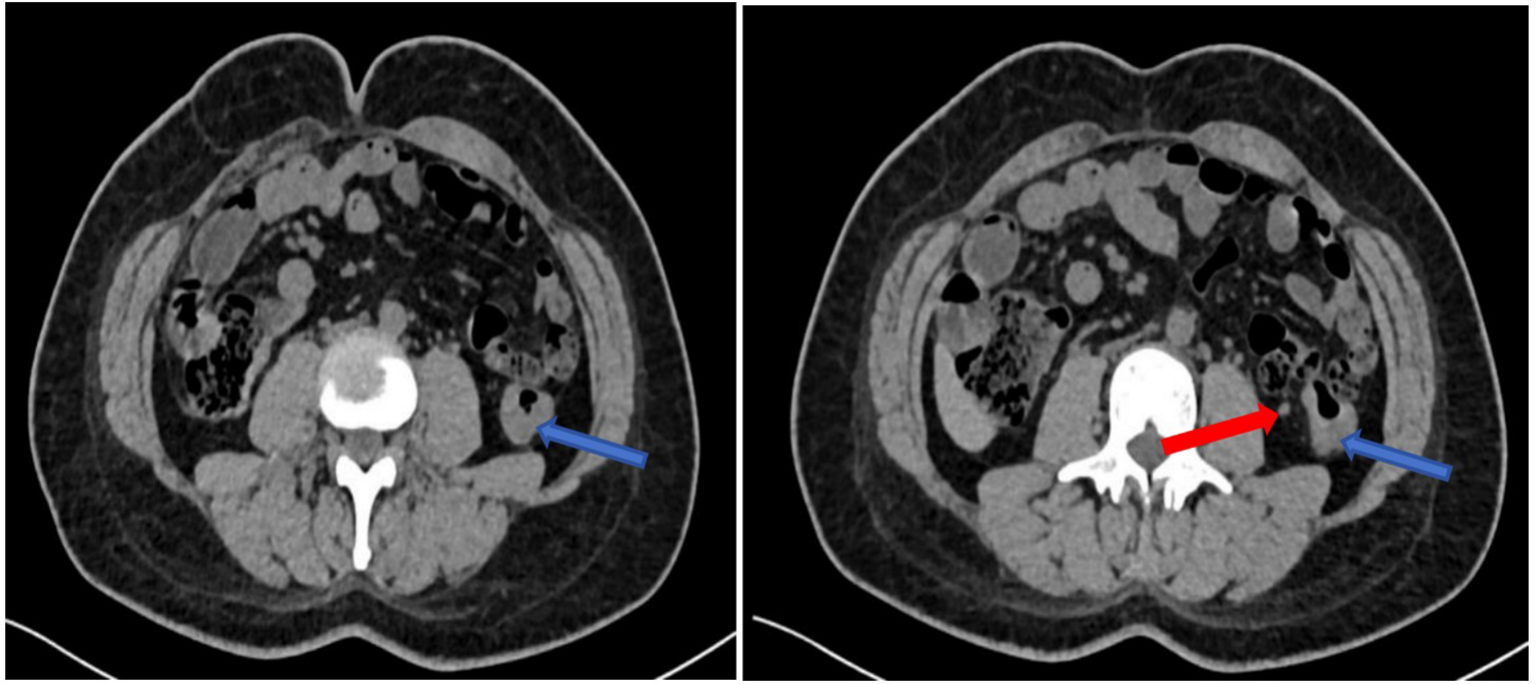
Images from the first CT exam on Feb 22, 2024, with red arrow indicating enlarged lymph nodes and blue arrows marking the tumor site.
